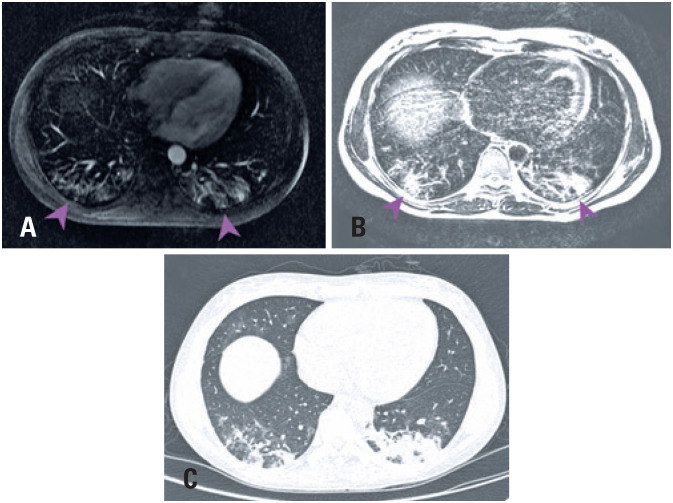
(A) axial upper abdominal computed tomography in T2 sequence ground-opacities showing subpleural and posterior predominance, suspected by COVID-19; (B) changes in the frame of the same resonance T2-weighted image, making findings more evident, similar to the one expected in computed tomography; (C) chest computed tomography of the patient at the same level showing a great similarity featured changes in magnetic resonance cuts
